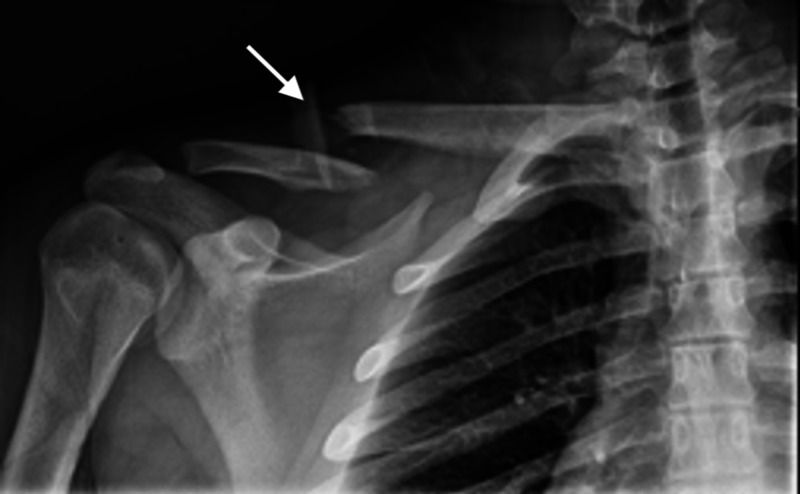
Clavicle fracture radiograph
